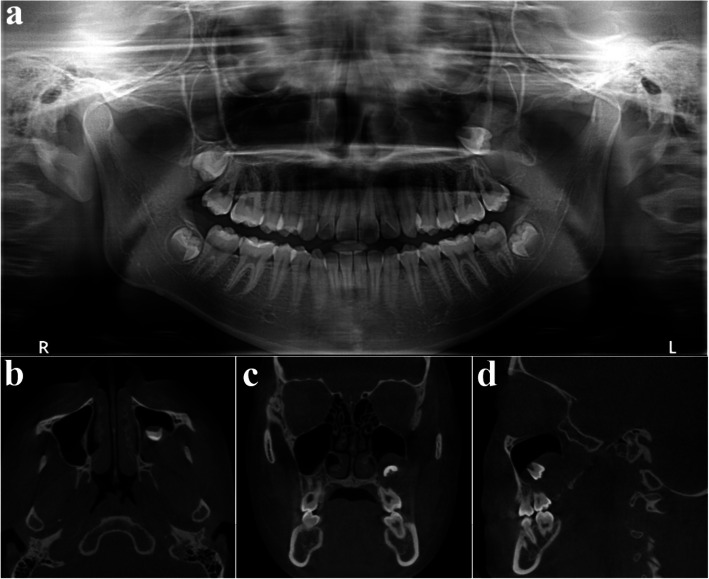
Preoperative radiographic images of a 15-year-old female patient with odontogenic keratocyst (OKC) involving an impacted left upper third molar, (a) panoramic radiograph, (b) CBCT, axial view, (c) CBCT, coronal view, (d) CBCT, sagittal view
